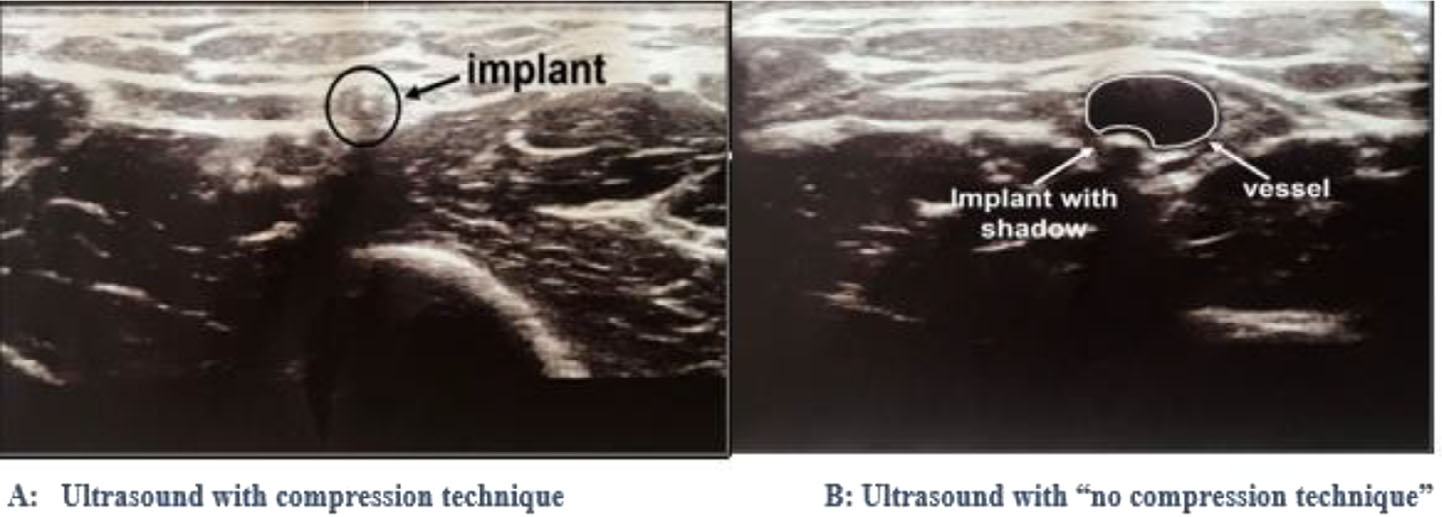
Ultrasound findings of Nexplanon with (A) “compression” and (B) “no compression” technique [30].
